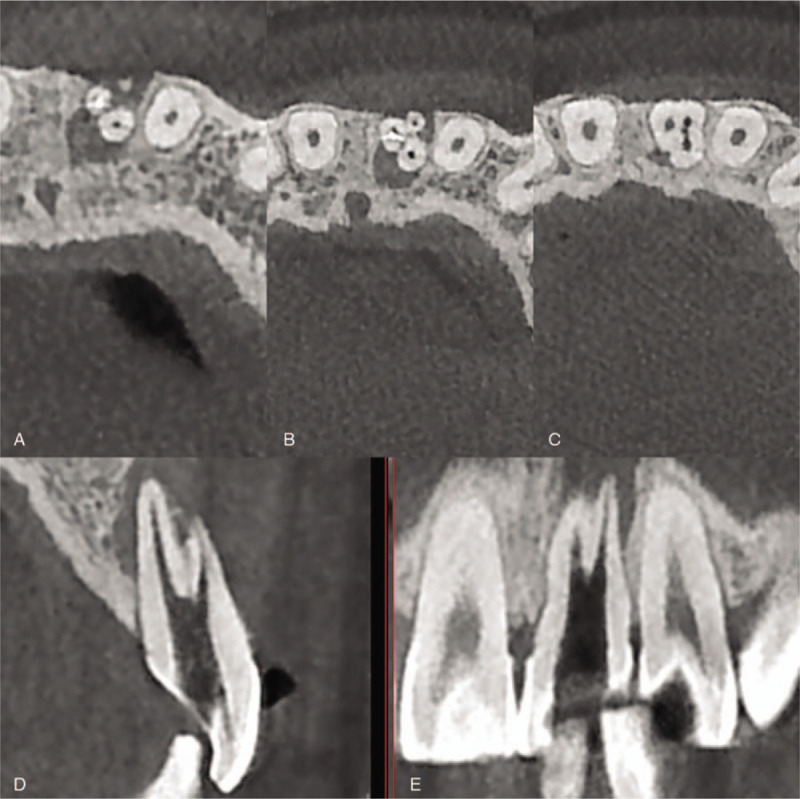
The cone-beam computed tomography (CBCT) scan of root canals, which distanced from root apex respectively (A) 3 mm, (B) 6 mm, and (C) 9 mm; (D) the sagittal view and (E) axial view of CBCT scan.
